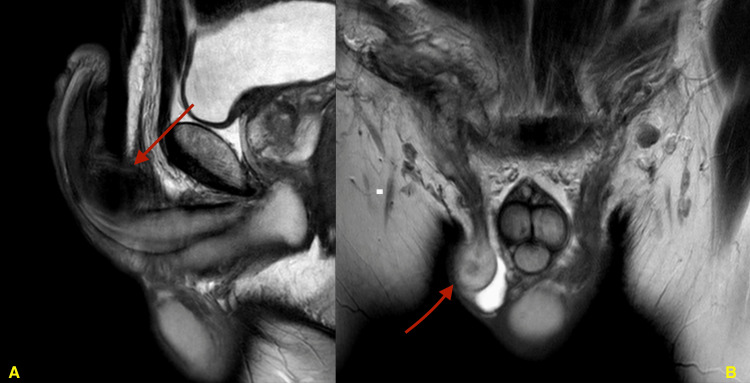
(A) Sagittal view of the MRI showing a penile fracture. (B) Coronal view of the MRI showing a right undescended testicle. MRI, magnetic resonance imaging
